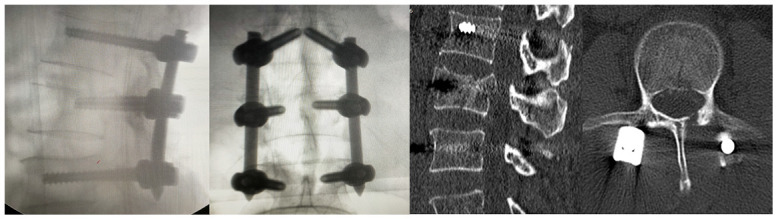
Postoperative X-rays and CT examination of the patient.
